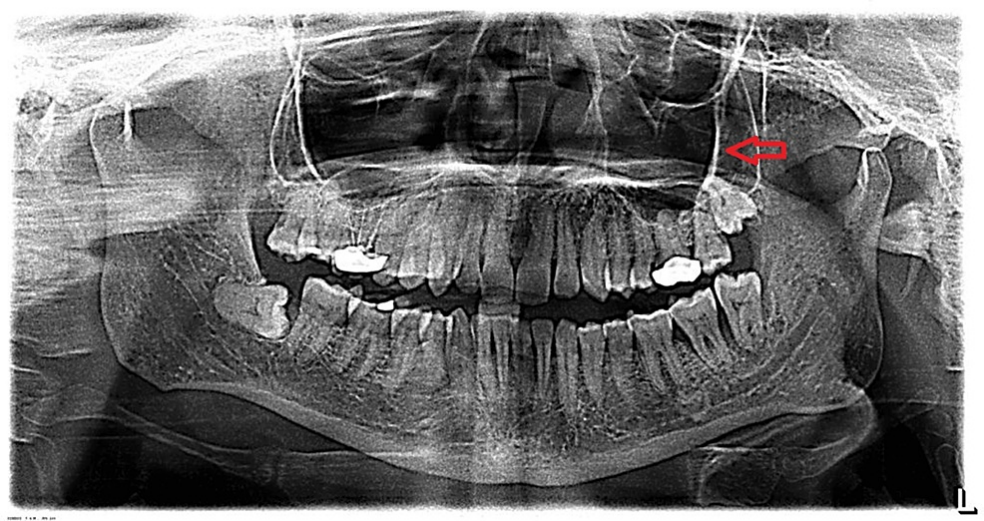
Panoramic x-ray shows impacted left maxillary third molar which is closely related to the maxillary sinus
